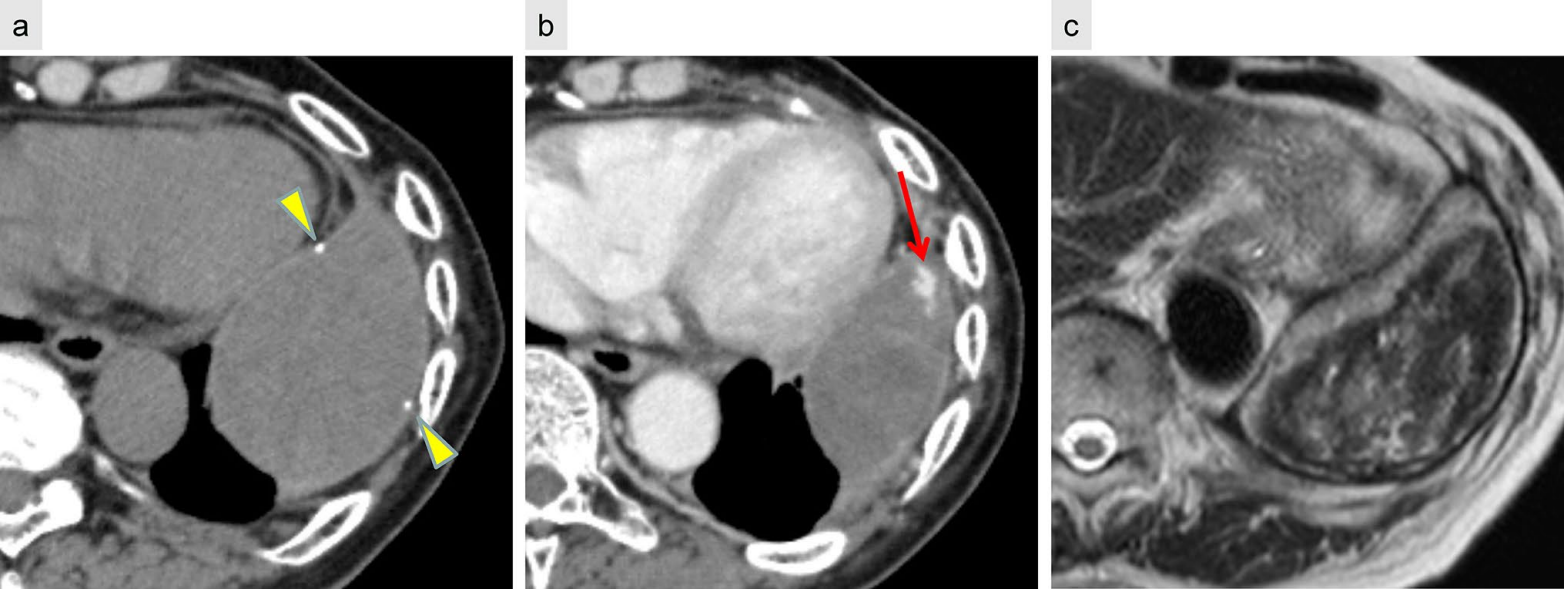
Chronic expanding hematoma. Five years following left lower lobectomy, non-contrast CT (a) reveals a mass with a focal calcified component (arrowhead) within the capsule. Contrast-enhanced CT (b) shows a small nodular enhancement at the lesion margin (arrow). T2-weighted MRI (c) demonstrates a mosaic pattern with areas of high and low signal intensity, characteristic of the “mosaic sign”
